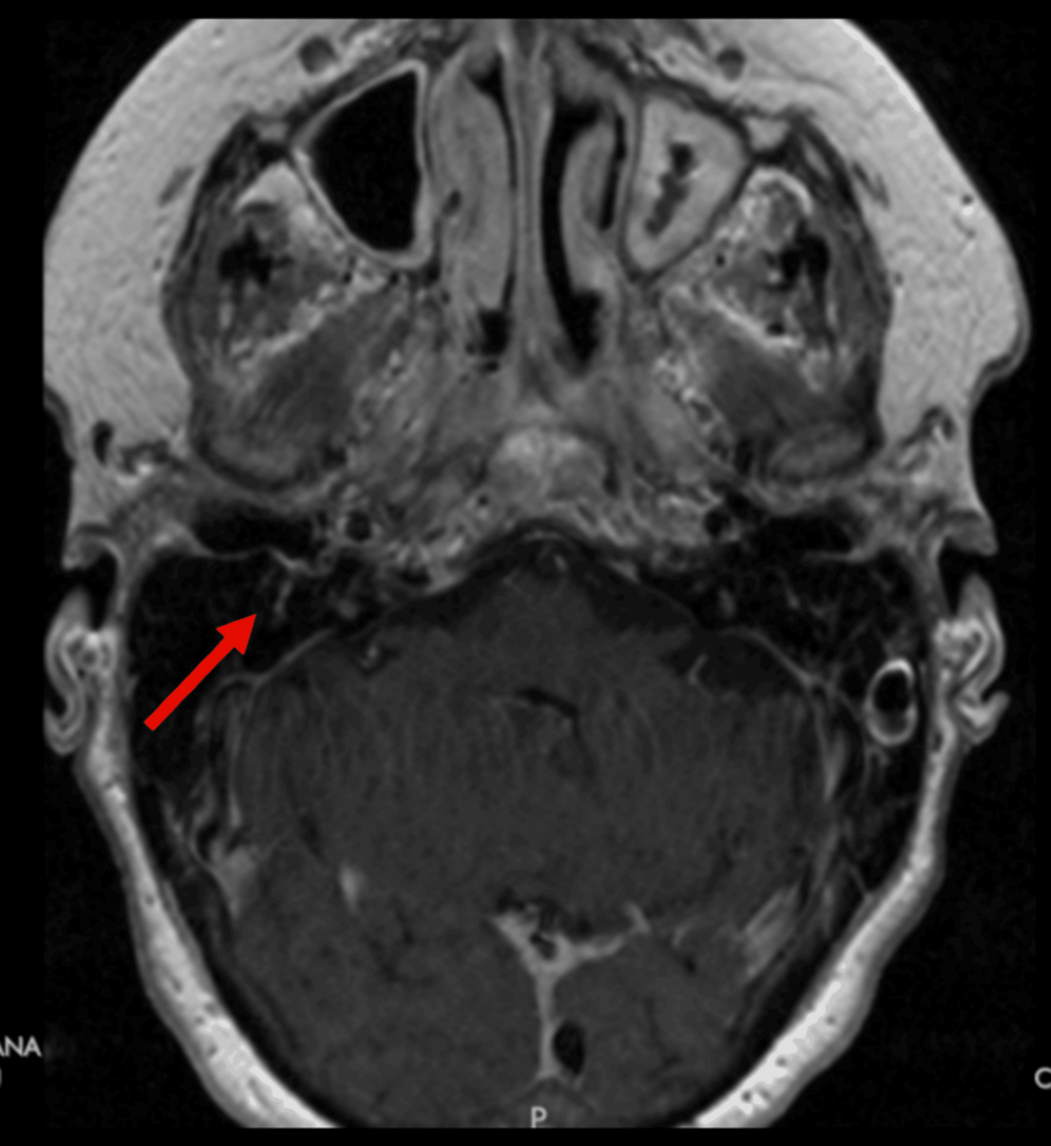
Axial post-contrast MRI of the brain demonstrating enhancement of the right cranial nerve VII (facial nerve) (arrow), consistent with an inflammatory process. No corresponding enhancement is noted on the left. MRI, Magnetic Resonance Imaging
